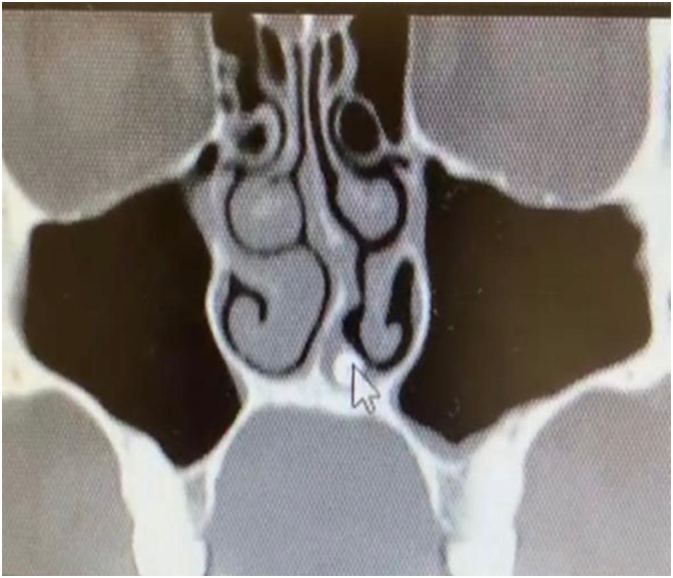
CT scan coronal cut showing the nasal tooth.
